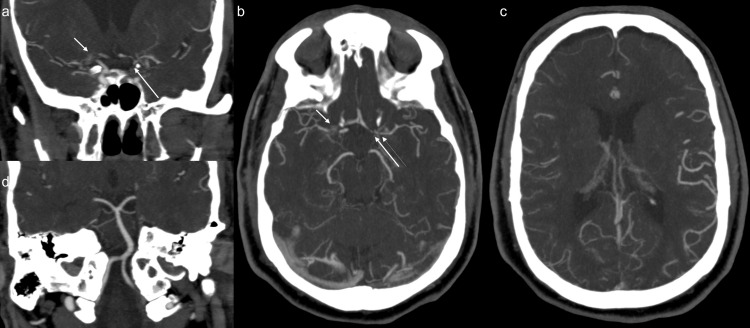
CTA head maximum intensity projection (MIP) shows occlusion and multivessel stenosis of the anterior circulation, with widely patent posterior circulation. (a) CTA coronal MIP shows occlusion of right internal carotid artery (ICA) terminus and origins of right anterior cerebral artery (ACA) and middle cerebral artery (MCA) (short arrow), and severe stenosis of supraclinoid portion of the left ICA (long arrow). (b) CTA axial MIP shows occlusion of right ICA terminus and origins of right ACA and MCA (short arrow), and severe stenosis of A1 segment of left ACA (long thick arrow), severe narrowing of the origin (long thin arrow) of left MCA. In addition, there is severe stenosis of proximal M2 branches on the right and moderate narrowing of the distal M1 and proximal M2 branches on the left. (c) CTA axial MIP shows diffuse narrowing and poor opacification of M3 and distal branches of right MCA. (d) CTA coronal MIP shows widely patent posterior circulation.
